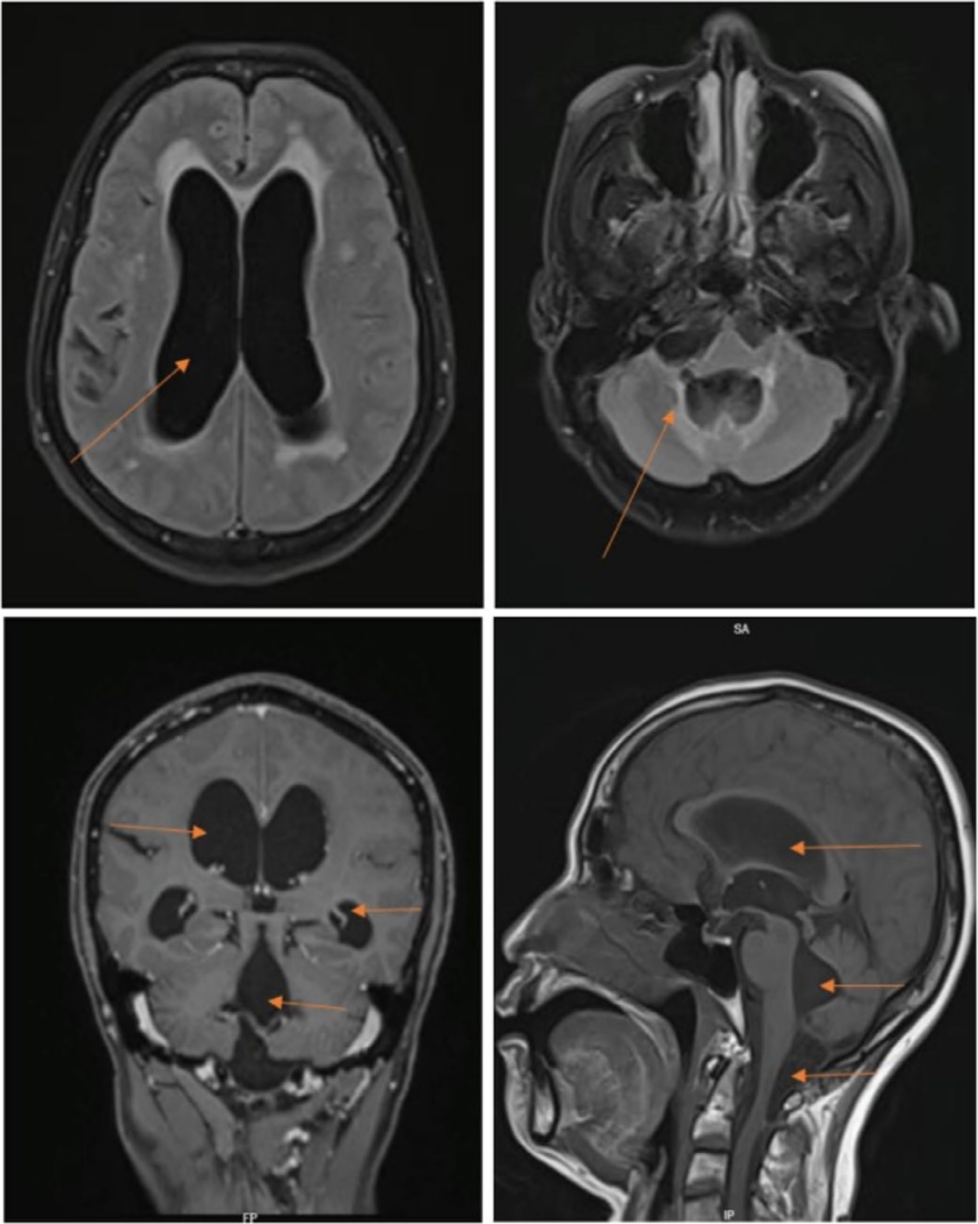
MRI of brain. Figure legend: (A) Flair showing hydrocephalus of the lateral ventricle. (B) Flair showing hydrocephalus of the fourth ventricle. (C) T1 coronal section post gadolinium showing hydrocephalus of all ventricles. (D) T1 sagittal section showing hydrocephalus of all ventricles (arrows showing dilated ventricles).
